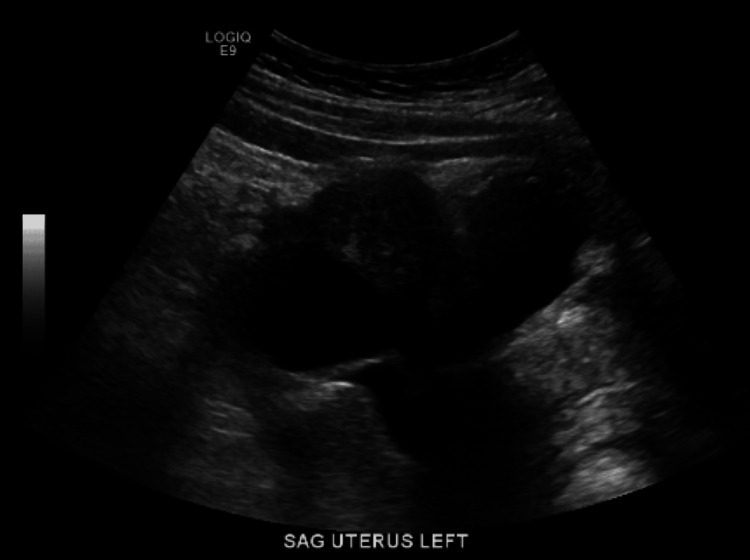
Ultrasound sagittal view of the uterus This transvaginal ultrasound (TVUS) sagittal view shows the uterus with a tiny cystic focus in the fundal endometrium. The radiology report stated that this may represent an early intrauterine gestational sac. However, pseudogestational sac and spontaneous abortion in progress or occult ectopic pregnancy could not be excluded, according to the radiology report.
